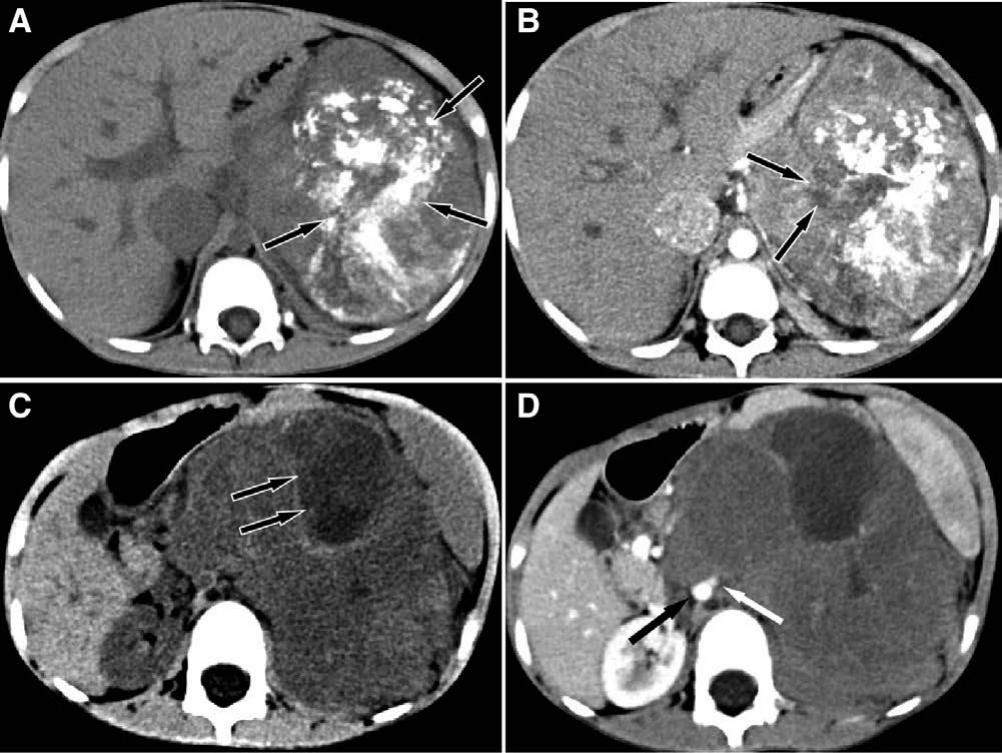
A 9-year-old girl with pathologically confirmed Wilms tumor, and CT showed a huge soft tissue mass in the left retroperitoneum with multiple calcifications (A, black arrows). Contrast-enhanced scans showed that the mass was heterogeneously enhanced, with low-density and non-enhancing necrotic areas (B, black arrows). A 5-year-old male patient with pathologically confirmed renal neuroblastoma, and CT showed a huge, superficially foliated, iso-soft-tissue density mass in the left kidney, and a large low-density cystic area was seen in the left kidney (C, black arrows). Contrast-enhanced scan showed mild enhancement of the lesion, and the mass grew around the abdominal aorta (D, black arrow) and left renal artery (white arrow). CT = computed tomography.
